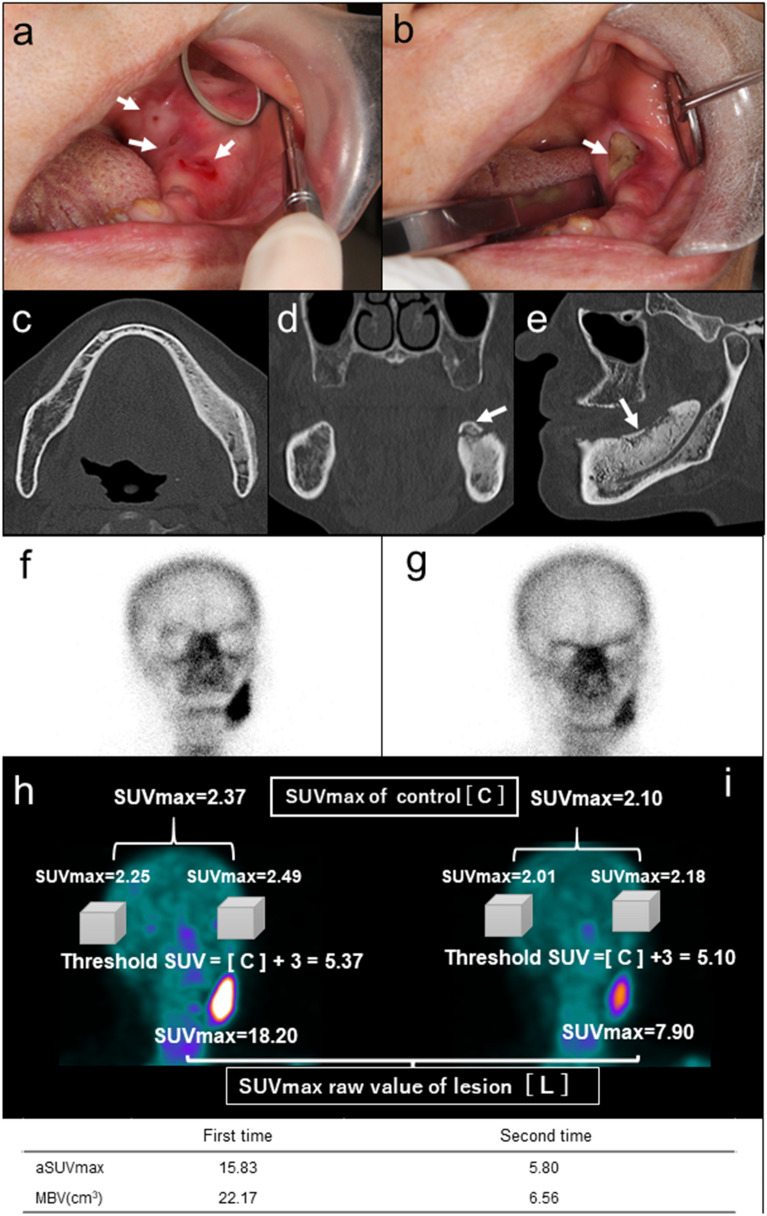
A representative case (patient 8), where there was intense swelling with redness in the left lower gingiva and discharge of pus from several fistulas at the baseline (*arrows*) (a). After anti-inflammatory therapy, the gingival swelling improved. The unification of fistula and exposure of the sequestrum was observed but pus was not observed (*arrow*) (b). CT at the baseline showed marked osteosclerotic changes of the lower left mandibular body and bone apposition on the outer surface of the cortical bone (c). Sequestrum was found in the coronal and oblique sagittal reconstruction images (*arrow*) (d, e). After pre-operative treatment, the sequestrum was removed by conservative surgical treatment and the patient reported no further recurrence. The 1st planar image showed a strong uptake in the lower left mandible (f) that remarkably decreased in the 2nd image (g). This 2nd image could not quantitatively represent the uptake intensity and volume. SPECT reconstructed the image by GI-BONE. The threshold for the volume of interest (VOI) was determined to be the value of the mean SUVmax of the right and left cranial bones plus three (1st/2nd round of SPECT) = (5.37/5.10). In the 1st SPECT imaging (h), adjusted SUVmax (aSUVmax) was caluculated SUVmax of lesion [L =18.20] - SUVmax of control [C =2.37] = 15.83. In the 2nd SPECT imaging (i), despite the short duration of 4.9 weeks, aSUVmax decreased sharply from 15.83 to 5.80. MBV also decreased sharply from 22.17 to 6.56 cm^3^.
